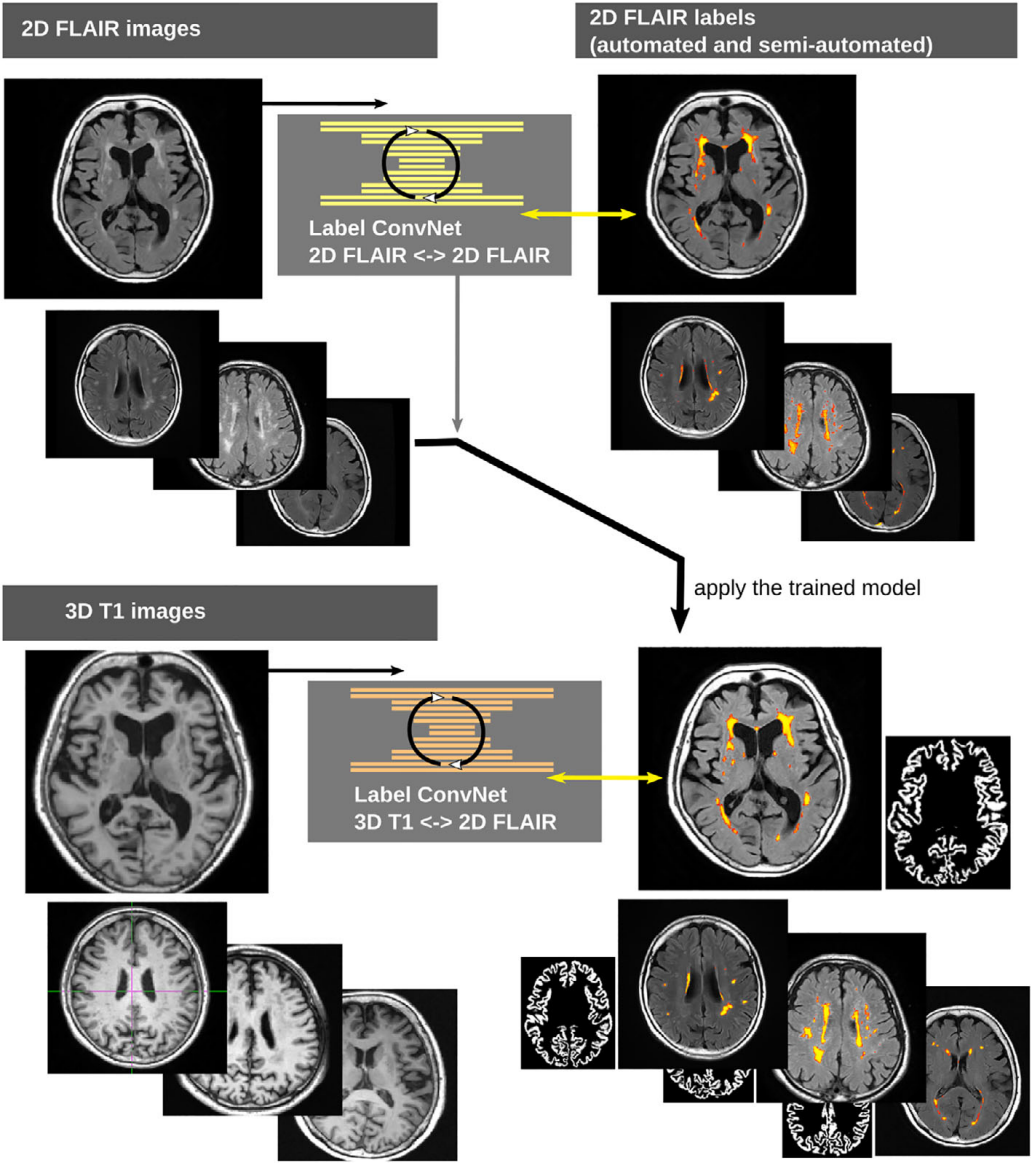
The two‐step learning process. The 2D FLAIR model (top) is trained to output a consistent FLAIR mask, initially based on the LST algorithm but with some further manual corrections. The T1 model (bottom) aims to learn a T1 WMH segmentation from those 2D‐FLAIR masks, learning across modality and resolution. It also learns a cortical ribbon as a secondary joint task. Both steps, including relevant preprocessing, use the same, single, training dataset.
